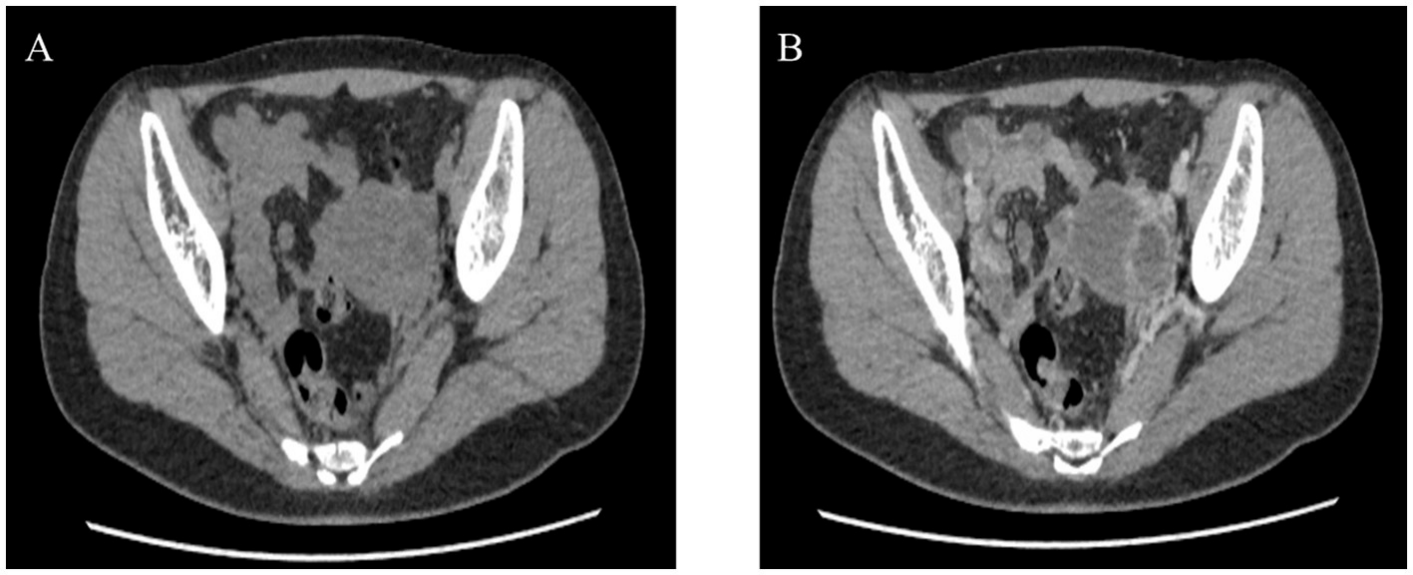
Preoperative CT, Heterotopic left kidney with congenital renal dysplasia and hydronephrosis; poorly visualized the left adnexa. (A) Plain CT. (B) Contrast-enhanced CT scan.
